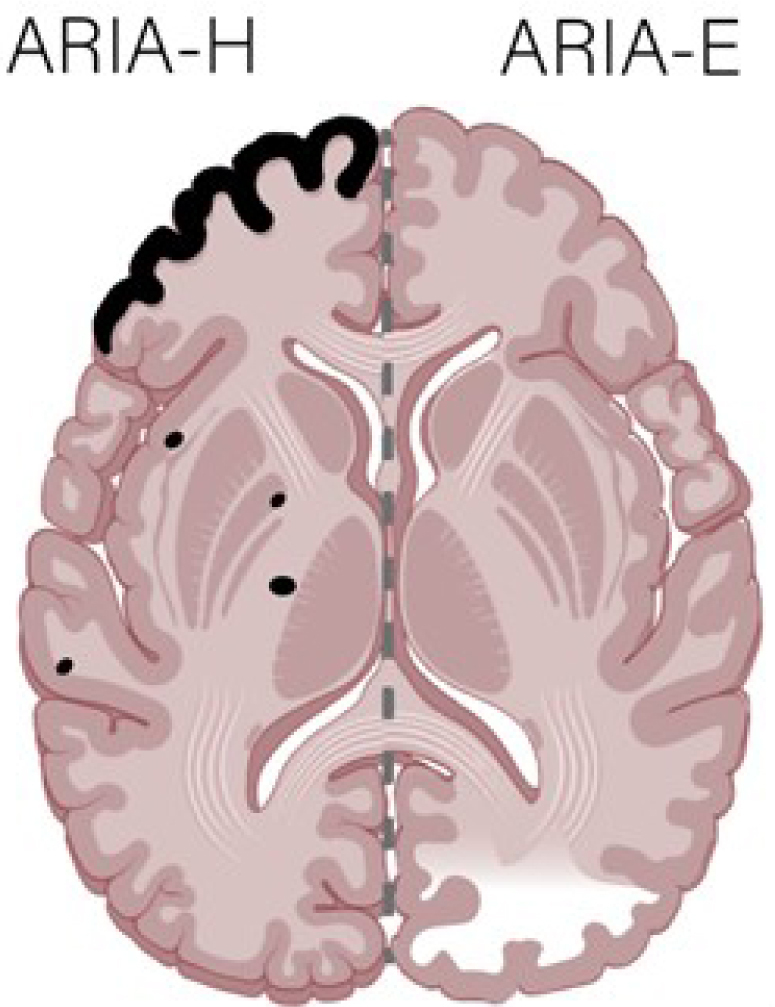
Amyloid-related imaging abnormalities with hemorrhage manifests in magnetic resonance scans as very low-intensity signals detected on gradient echo or susceptibility-weighted imaging magnetic resonance imaging sequences. Amyloid-related imaging abnormalities with edema commonly manifests as hyperintensities on fluid-attenuated inversion recovery or T2-weighted magnetic resonance imaging sequences with no restricted diffusion.
